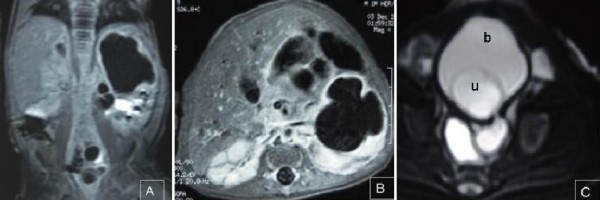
Uro-Magnetic Resonance Imaging showed a left ureteral duplication (A), hydro-ureternephrosis (A, B) and ureterocel (C), (b: bladder, u: ureterocel).
